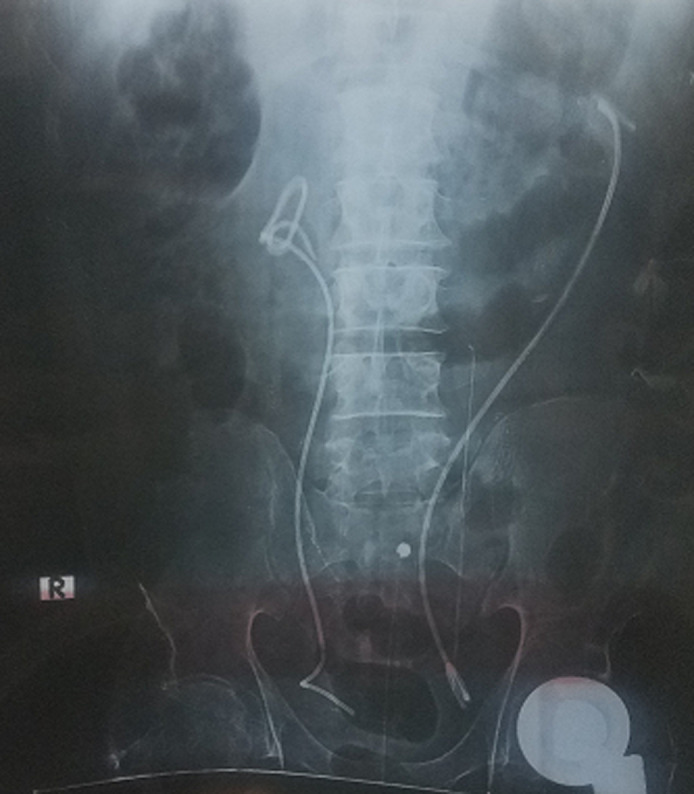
plain radiography X-ray showing both DJ stents at first insertion
